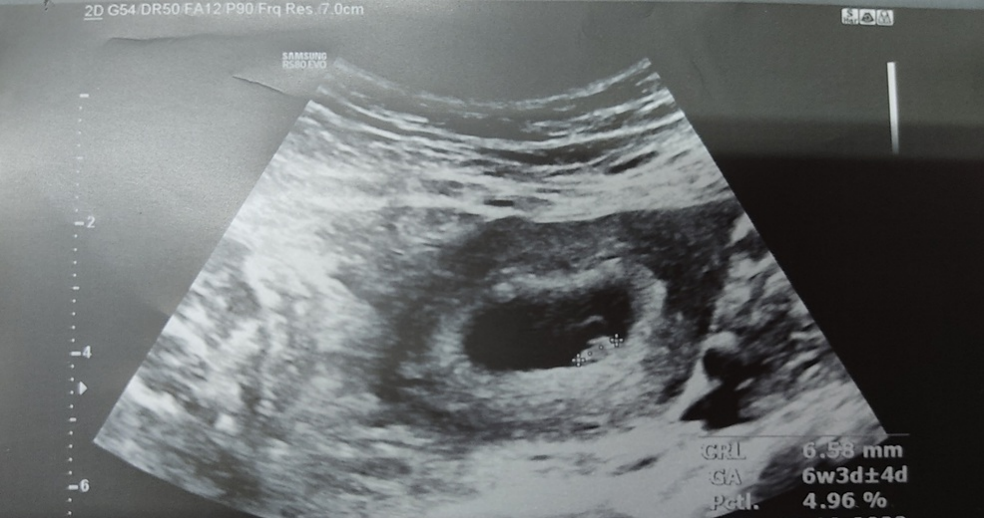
Ultrasonography image showing early intrauterine pregnancy
